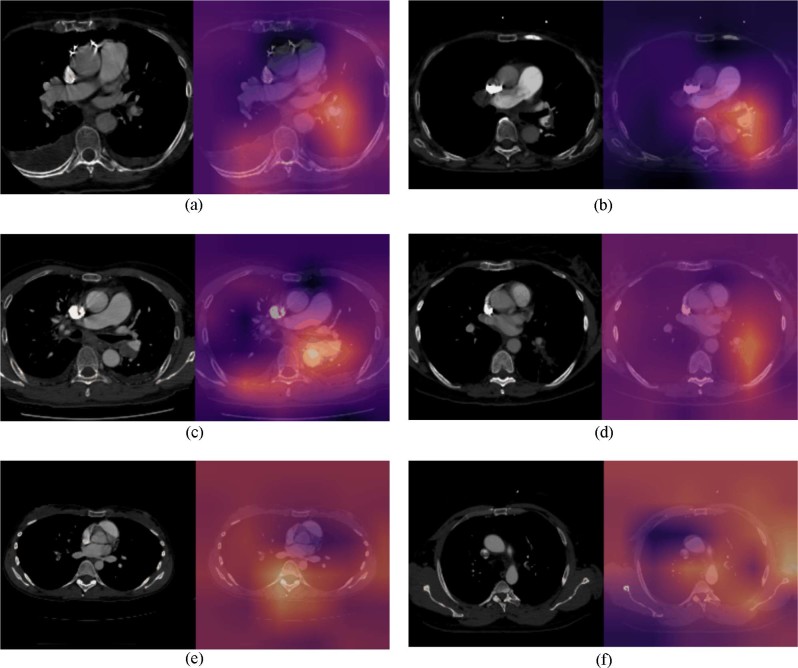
(Class Activation Maps): Class activation map (CAM) representation of true positive (Stanford (a) and Intermountain (b), false-positive (Stanford (c) and Intermountain (d) and false-negative samples (Stanford (e) and Intermountain (f)—axial contrast enhanced CT pulmonary angiogram (left) and CAM inferred by the model overlay with the scan (right). a (Stanford test set: true positive): (left) demonstrates a non-occlusive filling defect in a left lower pulmonary artery segmental branch that is correctly localized by the model as seen in the CAM image overlay (right). b (Intermountain test set: True Positive): (left) demonstrates a non-occlusive filling defect in a left main pulmonary artery that is correctly localized by the model as seen in the CAM image overlay (right). c (Stanford test set: false positive): left) demonstrates a large left hilar node adjacent to the pulmonary artery that is incorrectly labeled as PE by the model as seen in the CAM image overlay (right). d (Intermountain test set: false positive): (left) demonstrates an enlarged unopacfied left lower lobe pulmonary vein invaded by tumor that is incorrectly labeled as PE by the model as seen in the CAM image overlay (right). e (Stanford test set: false negative): (left) Pulmonary embolism in right middle lobe segmental branch that is missed by the model as seen in the CAM image overlay (right). f (Intermountain test set: False negative): (left) Pulmonary embolism in left upper lobe segmental branch that is missed by the model as seen in the CAM image overlay (right).
